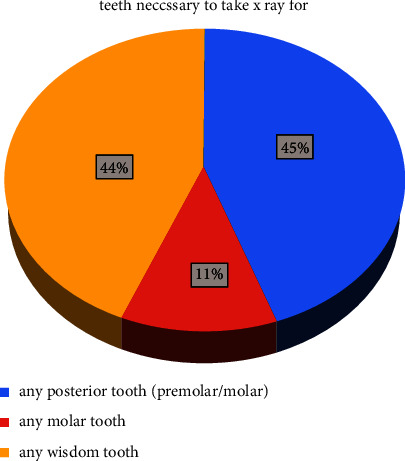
Teeth necessary to take X-ray for.
